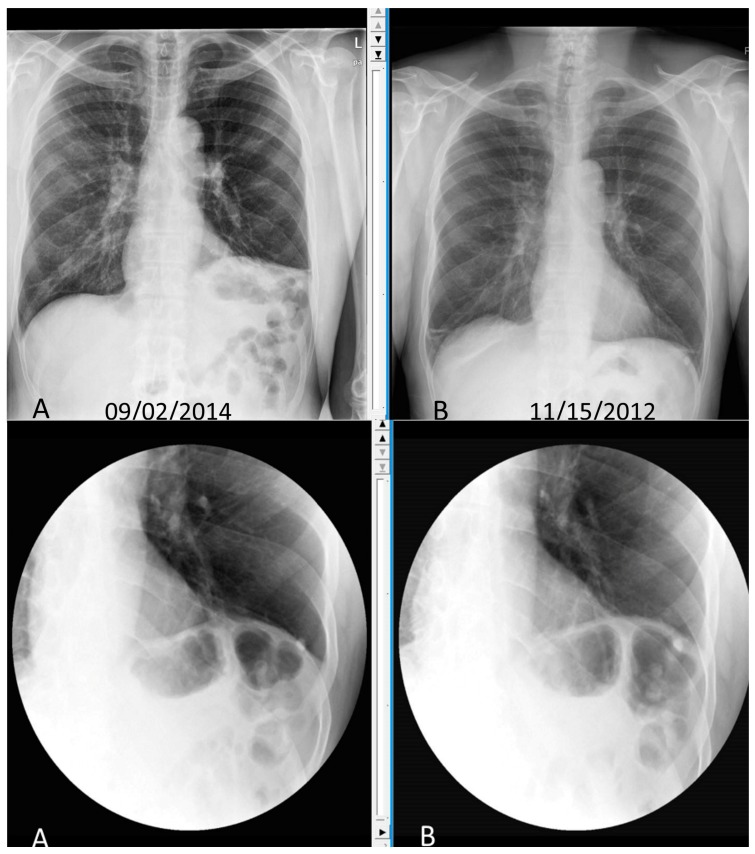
Chest X-ray and Static Sniff test of patient with cervical spondylosis leading to phrenic nerve compression and left diaphragmatic palsy. Top panel showing chest X-ray with elevated left diaphragm (A) compared to normal position on chest X-ray done 2 years back (B). Lower panel. Static Sniff test images in expiration (A) and after sniffing (B) showing no movement of diaphragm.
